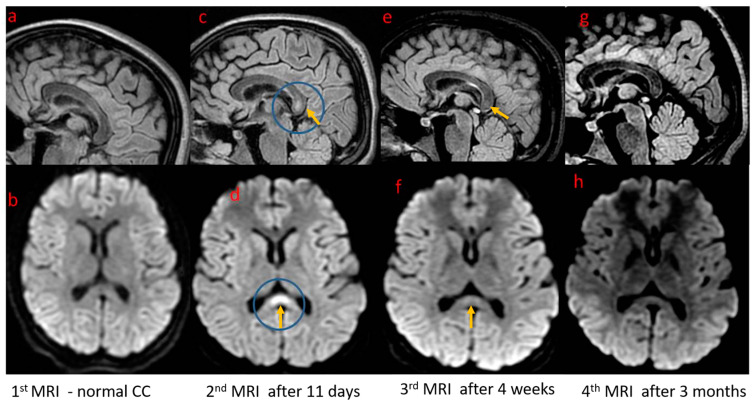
Brain MRI: T2 FLAIR sequence, in the sagittal plane (upper row) and DWI in the axial plane (lower row) showing the appearance and resolution of the abnormal signal in the corpus callosum (CC). (a,b) Initial MRI performed at the day of the onset of neurological symptoms (which precede the MRI one’s)—normal appearance of the CC. (c,d) Increased signal in the CC splenium with diffusion restriction 11 days after the onset of symptoms. (e,f) Partial resolution of changes after 1 month. (g,h) Further, nearly complete resolution after 3 months. MRI scans were performed using Artist 1.5 T GEM (General Electric, GE Healthcare, Milwaukee, WI, USA) according to the standard protocol comprising the most important sequences for CLOCC diagnosis: T2 Flair and DWI (diffusion-weighted imaging). Arrows and circle—corpus callosum.
